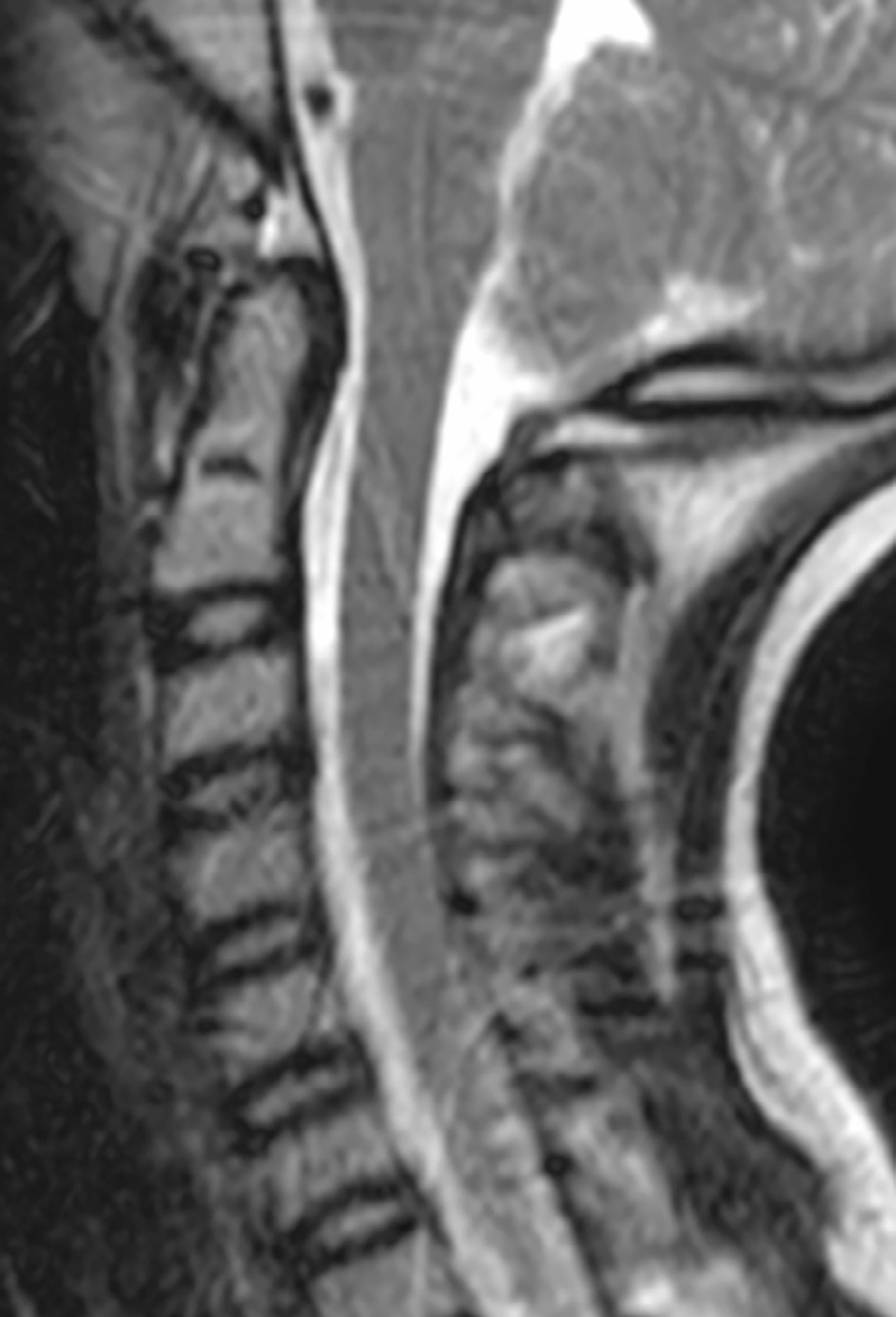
Sagittal *T*_2_-weighted static MR image of the cervical spine at neutral position showing normal alignment with no cord impingement or compression.
